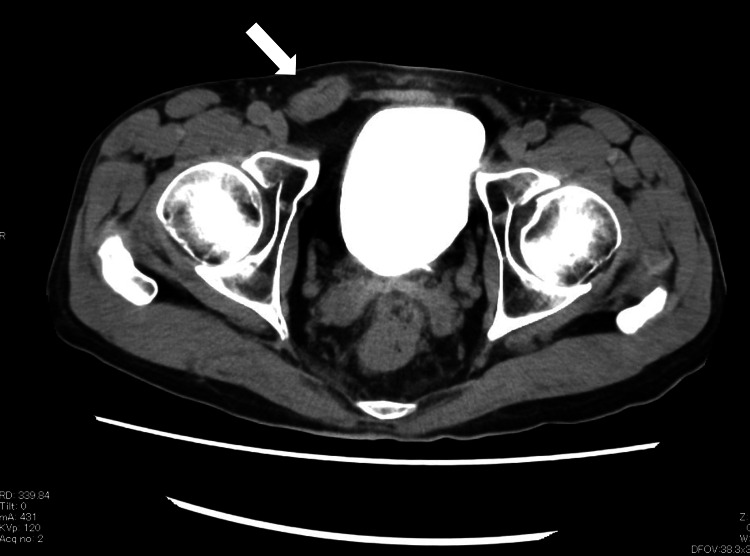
Post-reduction computed tomography An axial computed tomography image following manual reduction demonstrates localized edema along the spermatic cord in the right inguinal canal (white arrow), with no residual bowel incarceration.
